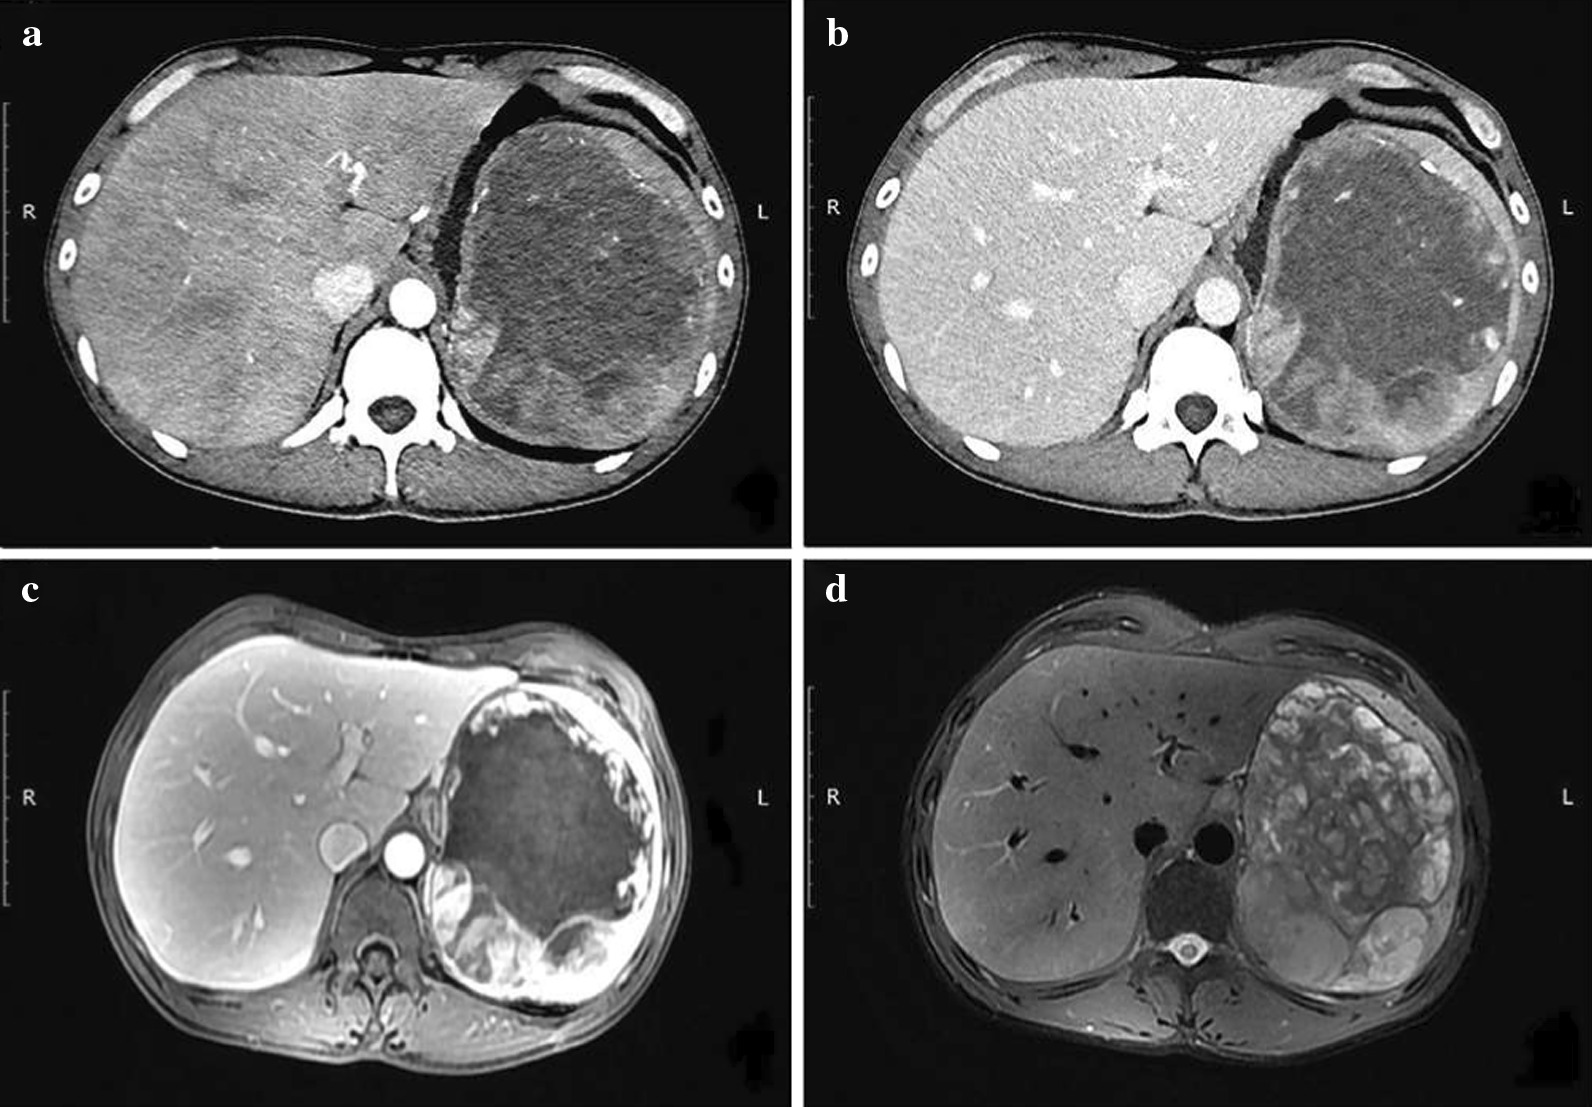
CT/ MRI-scan examinations. Enhanced CT in the upper abdomen: a, b were arterial and portal phases, respectively. Enhanced MRI in the upper abdomen: arterial phase of T2 was revealed in c. T1 fat-suppression images was shown in d
